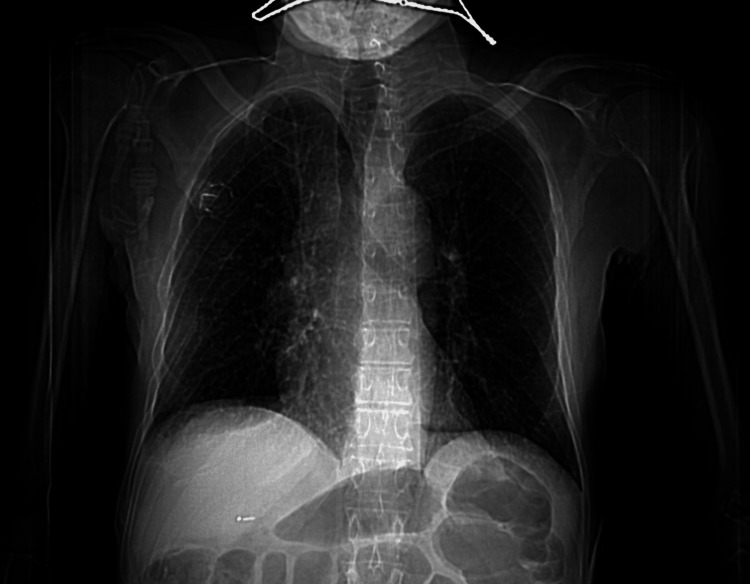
CT image demonstrating bulky mediastinal lymphadenopathy with mass effect on adjacent cardiac structures.
